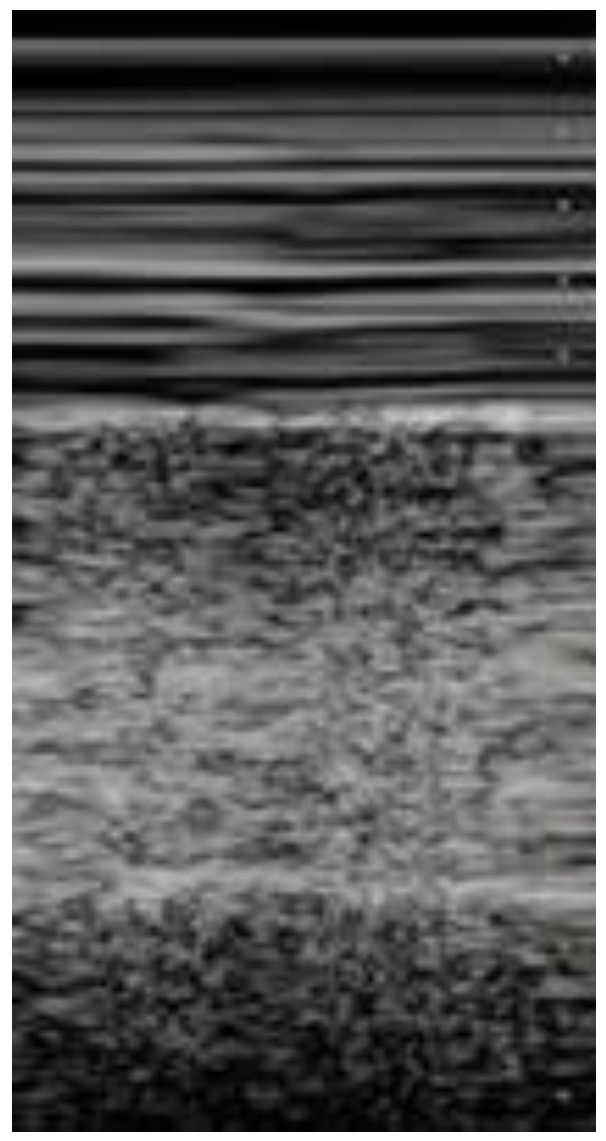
Lung ultrasonography, signs in motion mode (M-mode): seashore sign, indicative of the physiological sliding of the pleural line.
